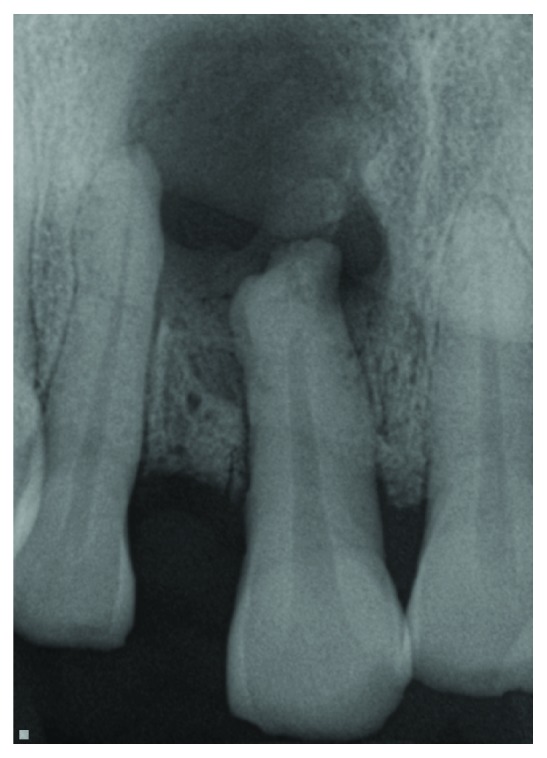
Periapical radiograph of large radiolucency associated with necrotic tooth #8.
